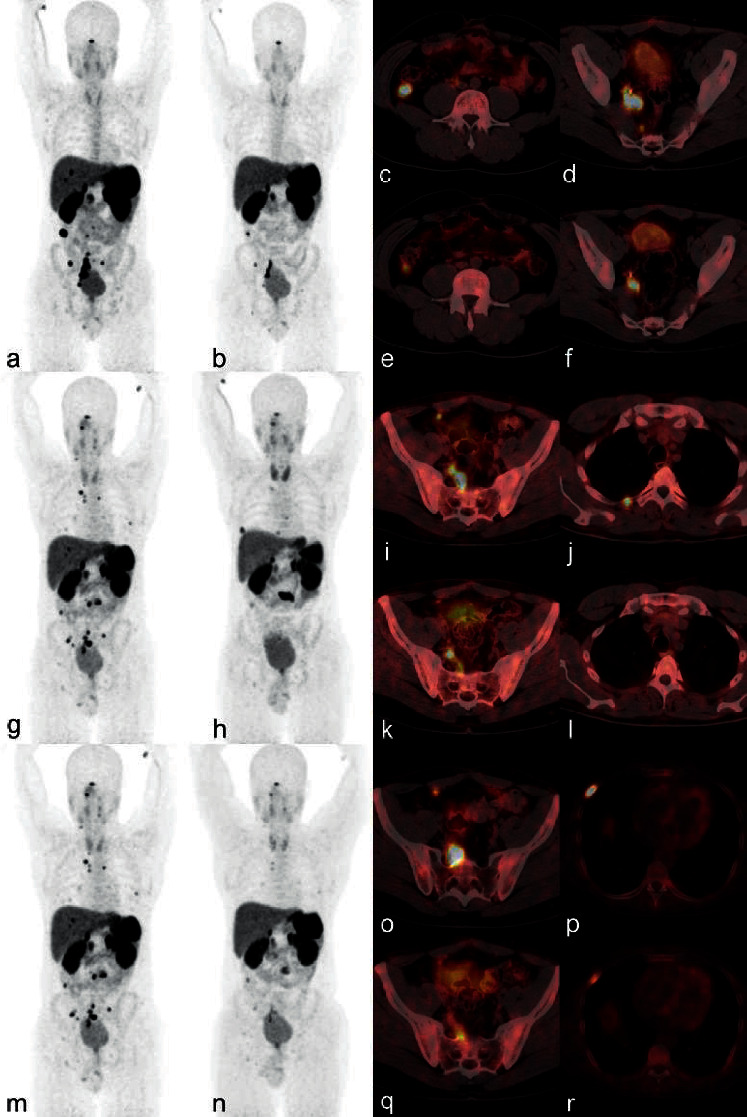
Example of the effect of the repeated tandem [^90^Y]Y/[^177^Lu]Lu-DOTATATE therapy: a 30-year-old man with a nonfunctional rectal G2 NET after surgery for primary tumours, with progression of the disease after 43 months. The [^68^Ga]Ga-DOTATATE PET/CT carried out for qualification to the PRRT showed increased uptake (higher than in the liver) in multiple, disseminated metastases. The patient received the initial PRRT, repeated PRRT, and re-repeated PRRT with the total accumulated injection activity of 29.6 GBq. The PFS rate after the I-PRRT, R-PRRT, and RR-PRRT was 35, 19, and 13 months, respectively. The disease progression after each therapy course was observed in different parts of the body. (a) [^68^Ga]Ga-DOTATATE PET MIP (maximum intensity projection) before the I-PRRT showing uptake in multiple metastases; (b) [^68^Ga]Ga-DOTATATE PET MIP after the I-PRRT showing partial response to the treatment; and axial fusion [^68^Ga]Ga-DOTATATE PET/CT (c, d) before and (e, f) after the I-PRRT. (g) [^68^Ga]Ga-DOTATATE PET MIP before the R-PRRT showing progression of the disease after the I-PRRT; (h) [^68^Ga]Ga-DOTATATE PET MIP after the R-PRRT showing partial response to the treatment; and axial fusion [^68^Ga]Ga-DOTATATE PET/CT (i, j) before and (k, l) after the R-PRRT. (m) [^68^Ga]Ga-DOTATATE PET MIP before the RR-PRRT showing progression of the disease after the R-PRRT; (n) [^68^Ga]Ga-DOTATATE PET MIP after the RR-PRRT showing partial response to the treatment; and axial fusion [^68^Ga]Ga-DOTATATE PET/CT (o, p) before and (q, r) after the RR-PRRT.
